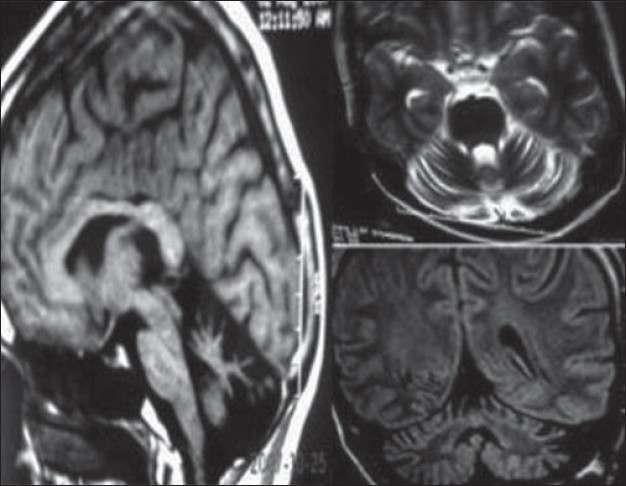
MRI images – isolated cerebellar atrophy
